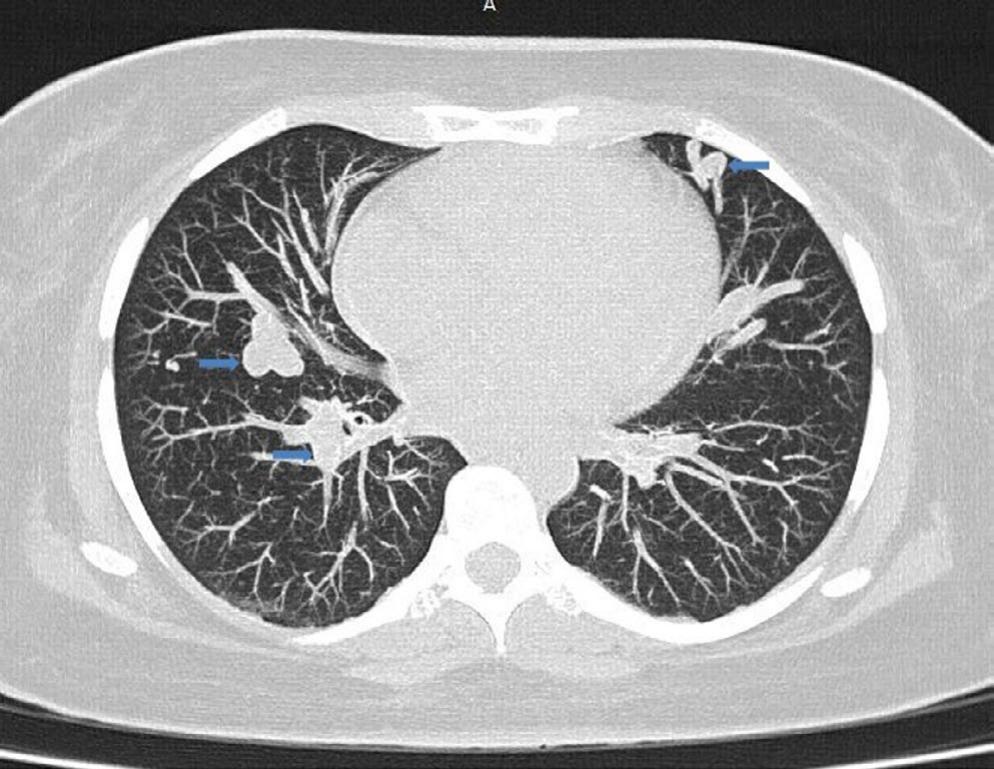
Parenchymal window chest CT scan with injection: multiple pulmonary arteriovenous malformations
